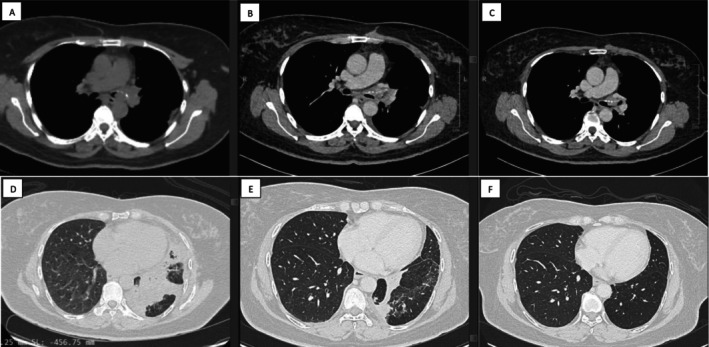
Computed tomography (CT) scan images of the thorax of the case. (A) At diagnosis, the adenoid cystic carcinoma mass (ACC) totally occluded the left main bronchus (LMB). (B) CT scan after two procedures showed some canalisation of the LMB. (C) CT scan after five procedures demonstrated a smaller mass and a linear passage at LMB. (D–F) The collapse consolidation of the left lower lobe improved with the dual debulking treatment from diagnosis, post‐second procedure and post‐five procedures.
